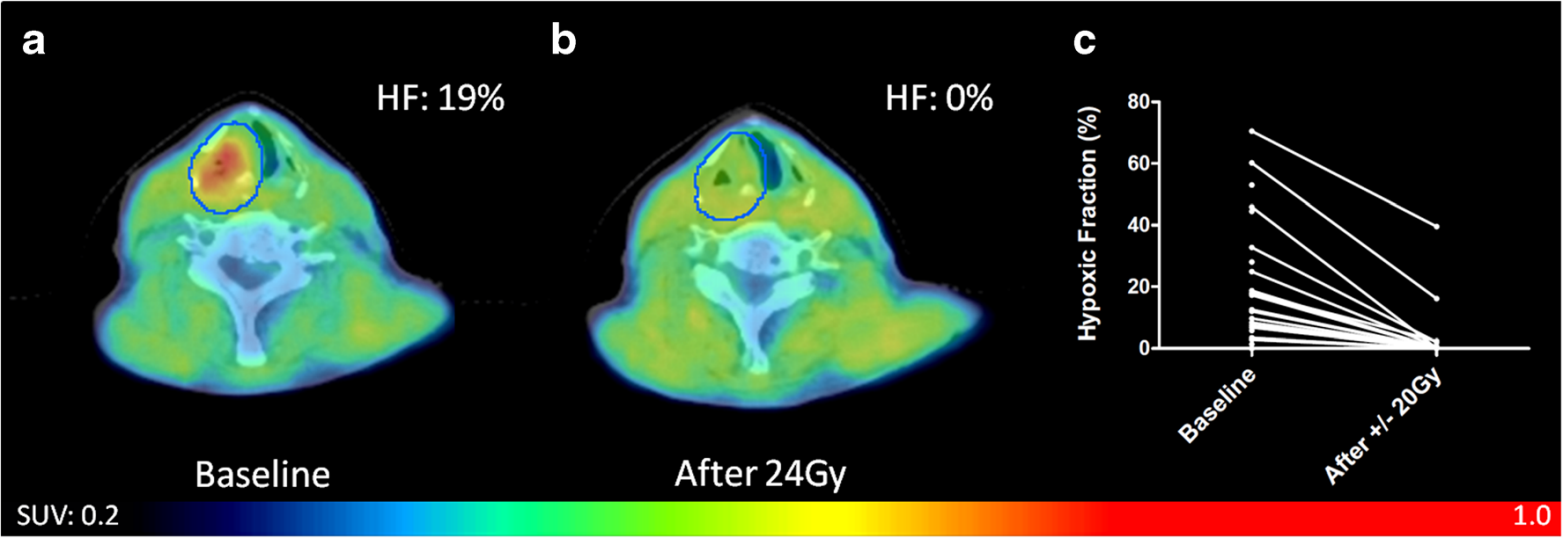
[^18^F]HX4 PET/CT scan of a patient with a T3N2bMx squamous cell carcinoma of the hypopharynx treated with cisplatin chemo-radiation. a: Scan with hypoxic primary tumour at baseline, b: decreased level of hypoxia during treatment and c: Calculated hypoxic fraction (HF) of all primary tumours and lymph nodes before and during treatment, significant decrease (*p* < 0.001)
